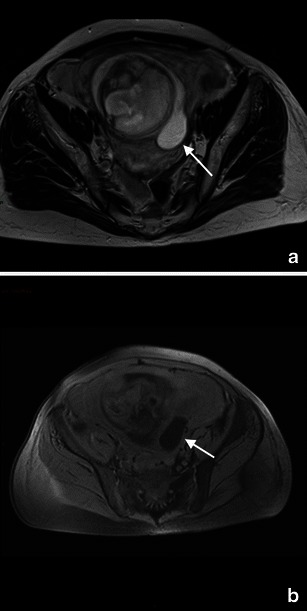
A 38-year-old woman was admitted at 26 weeks’ gestation presenting with vomiting and acute abdominal pain. Axial T2-weighted HASTE (a) and T1-weighted fat-saturated sequences (b) show posterior extravasation of amniotic fluid into a hernial sac that contains a small fluid level; these findings are suggestive of a sealed uterine rupture. Note the presence of haemoperitoneum
